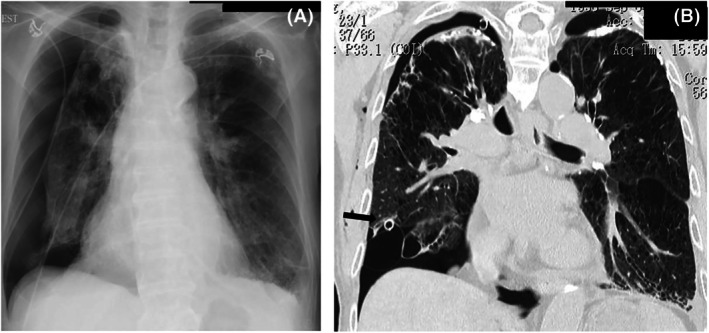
(A) Chest radiograph showing a right pneumothorax. (B) Coronal chest CT image demonstrating upper‐lobe emphysema and lower‐lobe fibrosis consistent with CPFE; chest tube in situ (arrow) with partial re‐expansion.
